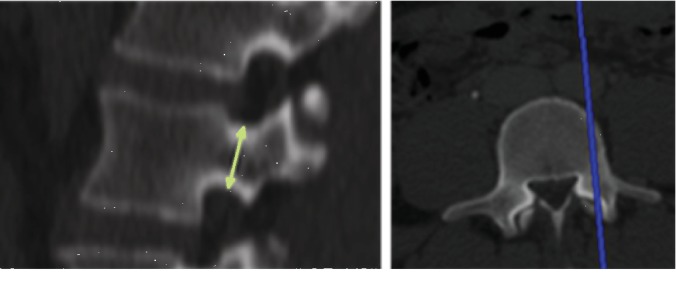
Measurement of pedicle height as conducted on a sagittal CT scan (a) at the middle of pedicle width (b).
